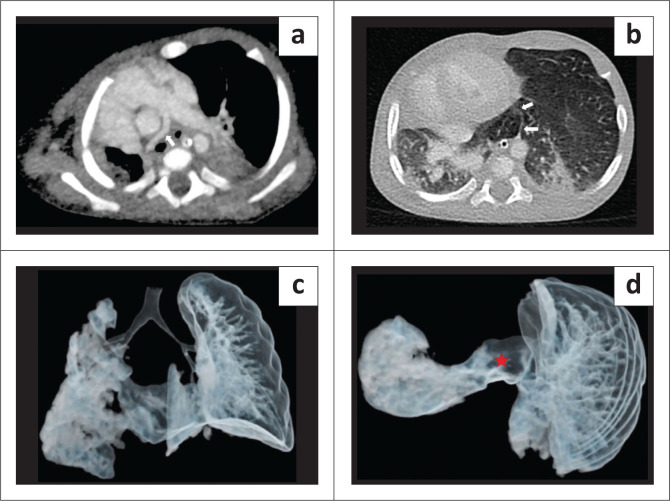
Pseudo-horseshoe lung in a 3-month-old male associated with right lung hypoplasia, anomalous unilateral single pulmonary vein and superimposed pulmonary infection. (a) Axial maximum intensity projection image of contrast enhanced CT chest (mediastinal window) shows the origin of the hypoplastic right pulmonary artery (arrow) from the main pulmonary artery. (b) Axial CT lung window image reveals fusion of the basal segments of the lower lobes with an intervening isthmic fissure (arrows). The isthmus of lung parenchyma is present posterior to the heart and anterior to the aorta and the oesophagus (as indicated by the nasogastric tube). An area of consolidation is present in the left lower lobe. (c and d) 3D volume rendered images demonstrate the fusion of the basal segments of both lungs via a parenchymal isthmus (red star) arising from the hypoplastic right lung.
